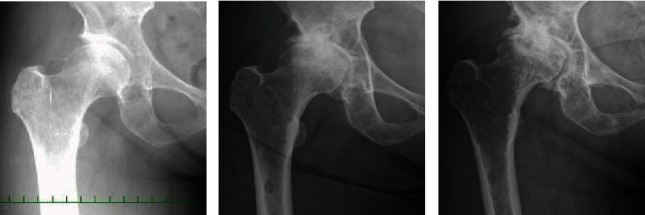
Plain radiographs, (a) 13 years prior to surgery, (b) 2 years prior to surgery, and (c) 1 month prior to surgery, reveal progression of arthritic changes including loss of joint space and femoral head cyst. Acetabular dysplasia with a center-edge angle of 13 degrees and sharp angle of 47 degrees, osteoarthritis with loss of joint space and bone cysts in the femoral head, and multiple myeloma with punched-out lesions are depicted.
